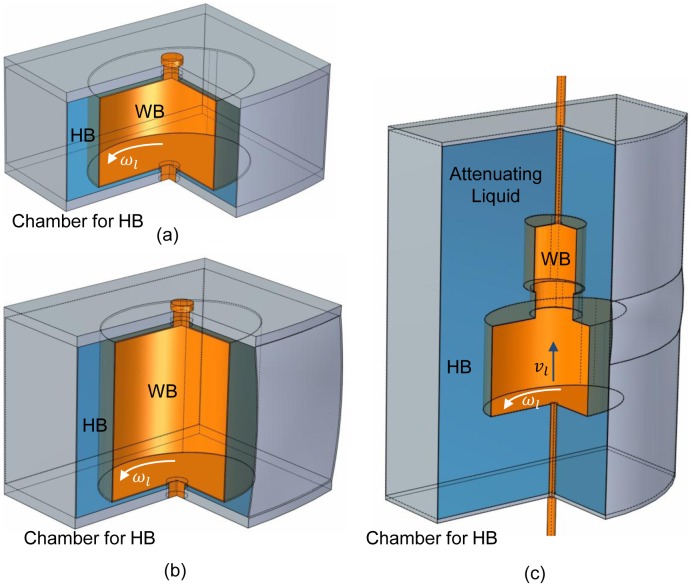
Exemplary designs of the dynamic bowtie without driving components. (a) A dynamic bowtie for fan-beam CT, (b) and (c) for cone-beam CT and spiral multi-slice CT respectively. The attenuating liquid for HB is
